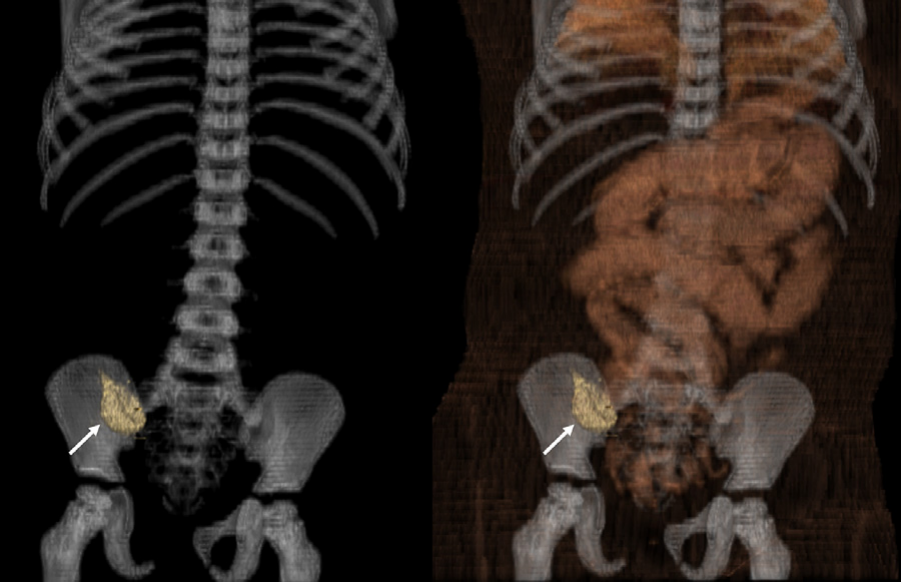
3D reconstructed computed tomography (CT) data, showing low-density lesion (white arrow).
